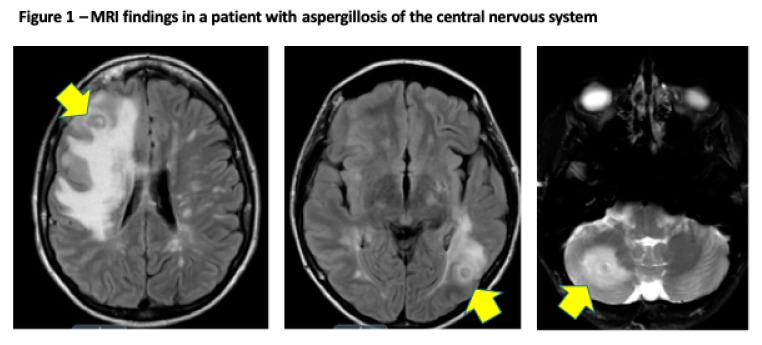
Proven cerebral aspergillosis in a 30-year old female diagnosed within 45 days after orthotopic liver transplantation. MR of the brain shows hyperintense T2 and FLAIR signal throughout the cerebral white matter, and deep gray nuclei structures with discrete ring enhancing lesions and surrounding edema seen in the left occipital lobe and right cerebellum (arrows).
